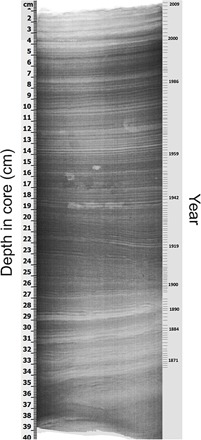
X-radiograph of sediment box core. Chronology was assigned by enumerating individual varve couplets. A bacterial mat 1 to 2 cm thick at the top of the core indicates that surficial sediments were intact.
